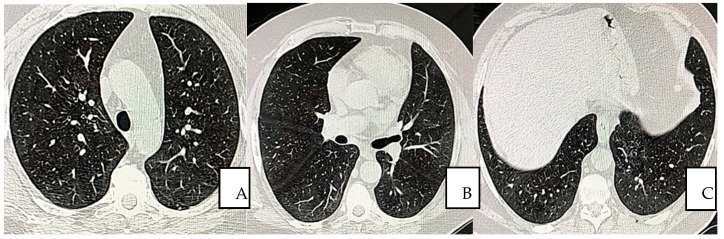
(A–C) Pulmonary non-contrast enhanced chest CT scan obtained on day 60 from symptom onset (16 February 2021), shows continued and completed resolution without residuals. Parenchymal, mediastinum, and peripheral (A,B), lower lobe I opacities and bands are not observed (A,B).
